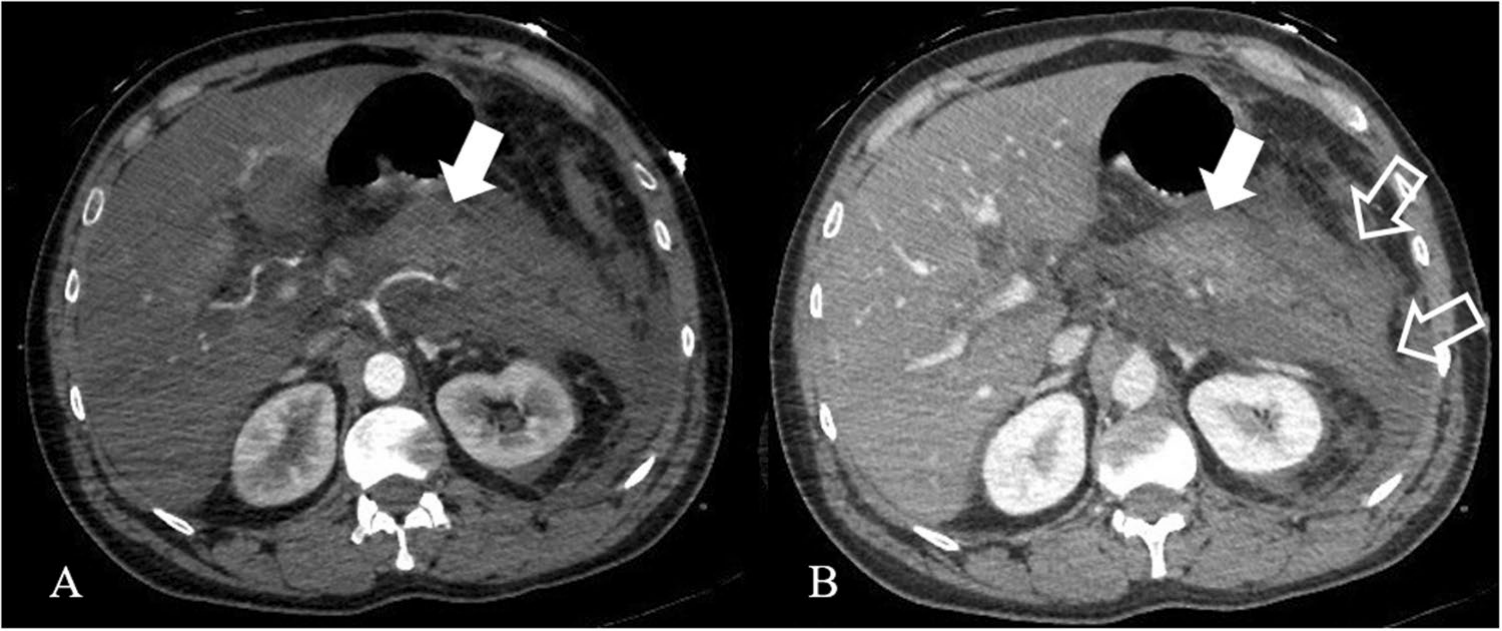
Necrotizing Pancreatitis in a 39 years-old man, with acute abdominal pain and sepsis. Axial contrast-enhanced CT scans during the arterial phase (a) and portal phase (b) show enlarged pancreas with poorly defined contours and decreased enhancement of the pancreatic parenchyma (arrows), surrounded by heterogeneous fluid collection (empty arrows in b)
